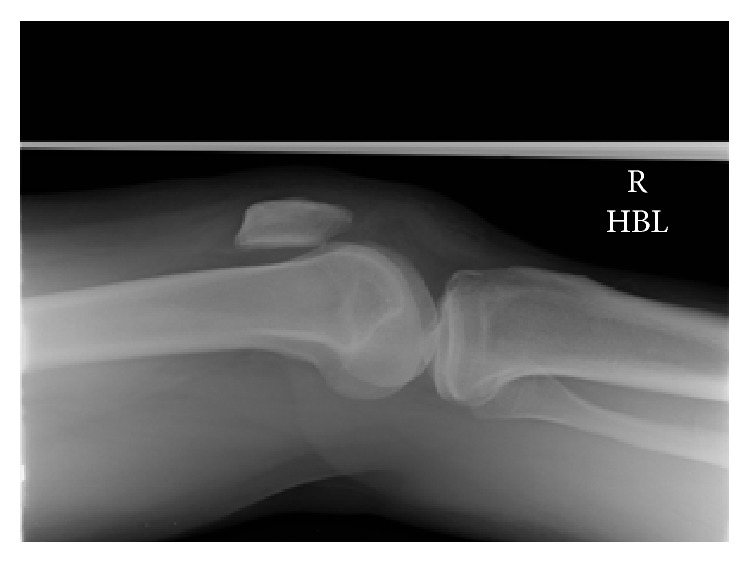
Lateral radiographs of right knee.
